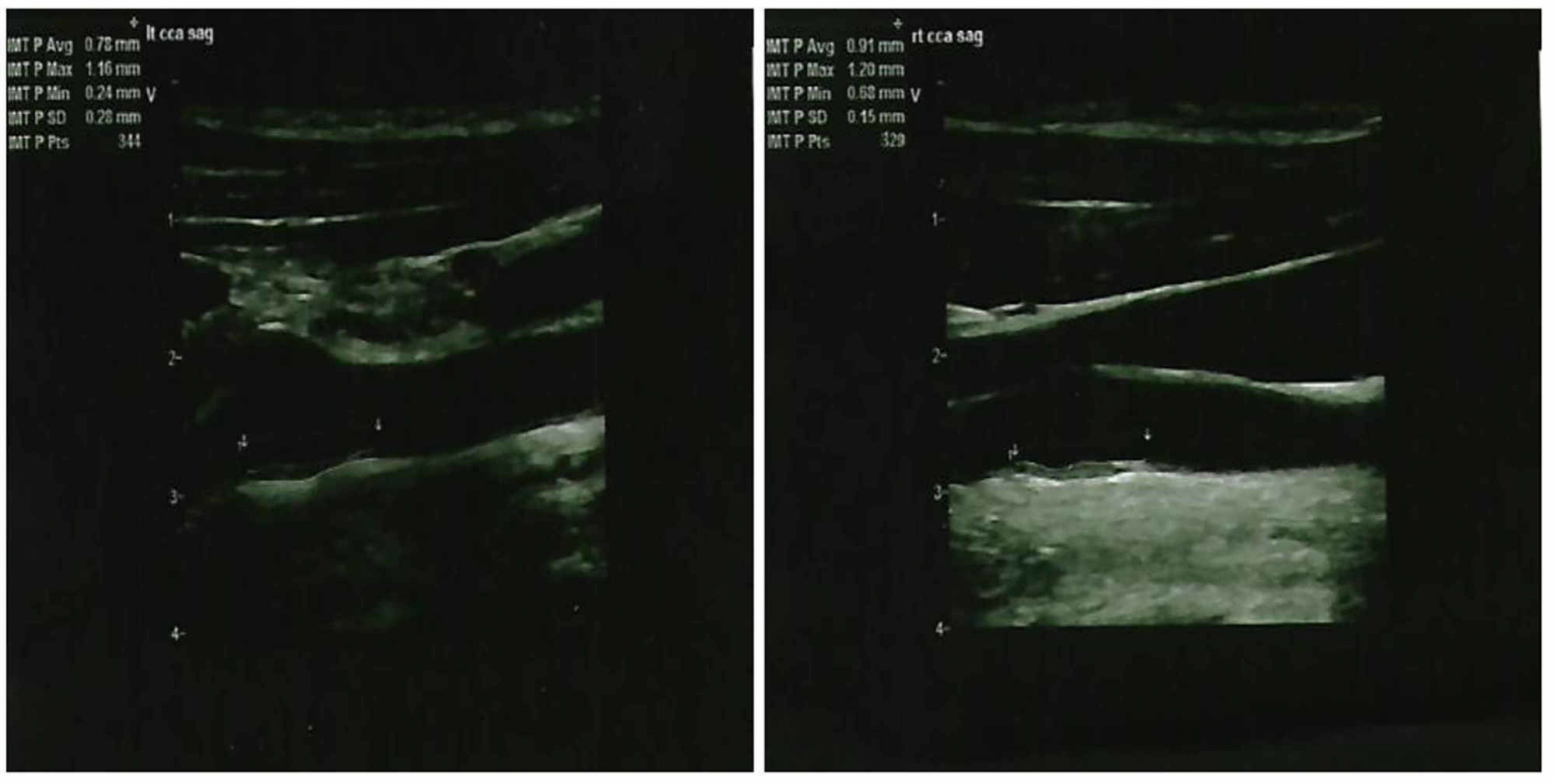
Ultrasound showing the left common carotid intima-media thickness (left panel) and right common carotid intima-media thickness (right panel) in 2017.
